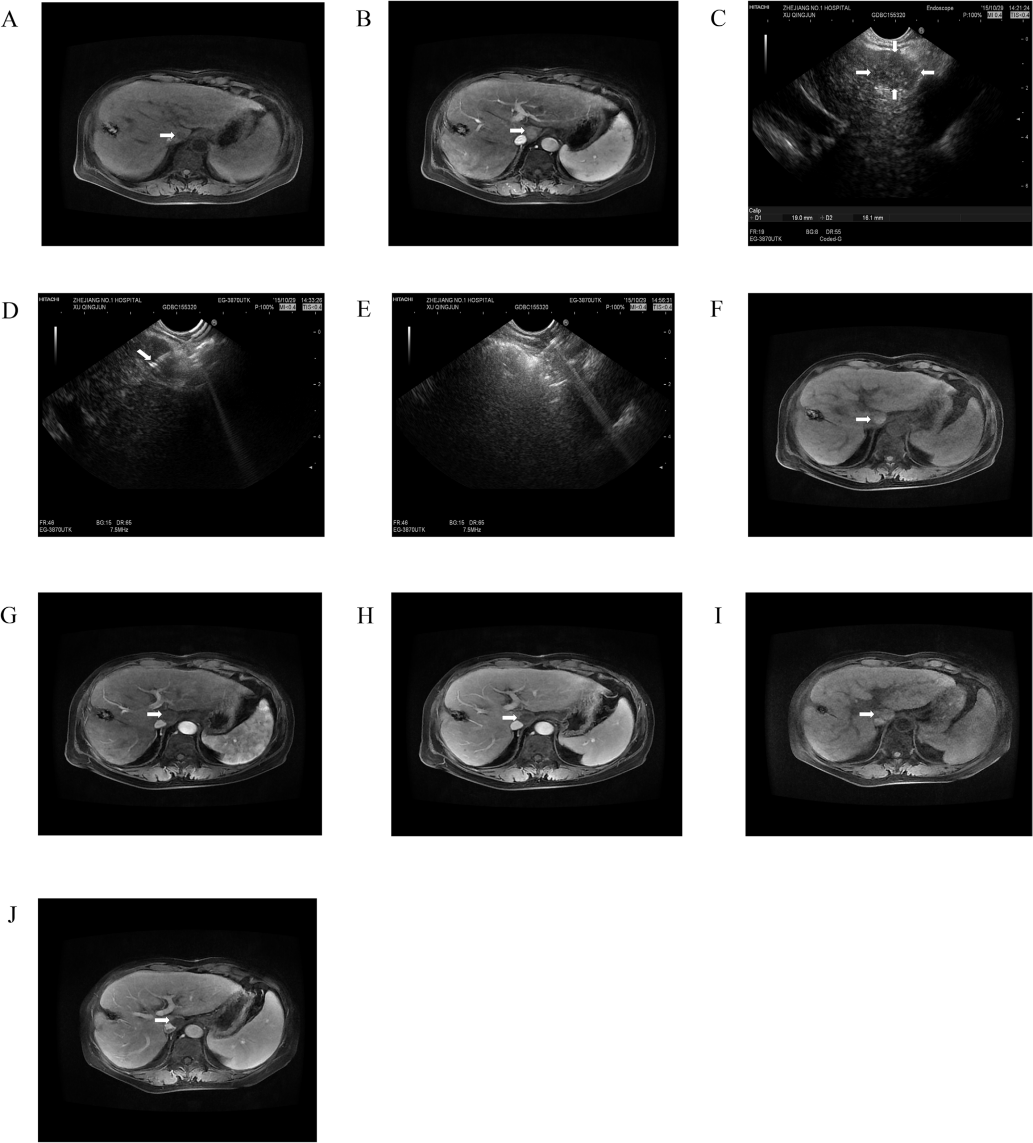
EUS-guided anhydrous ethanol ablation for lesions in liver caudate lobe. MRI imaging in liver caudate lobe showed a lesion of low T1 signal intensity (A) (arrowhead) and significant enhancement in arterial phase (B) (arrowhead). (C) at liver caudate lobe EUS scan showed a well-defined hypoechoic 1.9*1.6 cm lesion (arrows). (D) It indicated that 22G biopsy needle with EUS guidance was inserted into the lesion along with the needle sheath (arrowhead), and (E) diffuse increase in echogenicity covering the whole mass after the percutaneously punctured injection of anhydrous alcohol. After 1 month follow-up, MR scan was seen high T1-weighted signal intensity (F) (arrowhead) and no obvious enhancement during arterial (G) (arrowhead) and substance phase (H) (arrowhead). After 15 months follow-up, it showed high T1-weighted signal intensity (I) (arrowhead) and contrast material-enhanced MR images showed completed ablation without enhanced lesions (J) (arrowhead).
